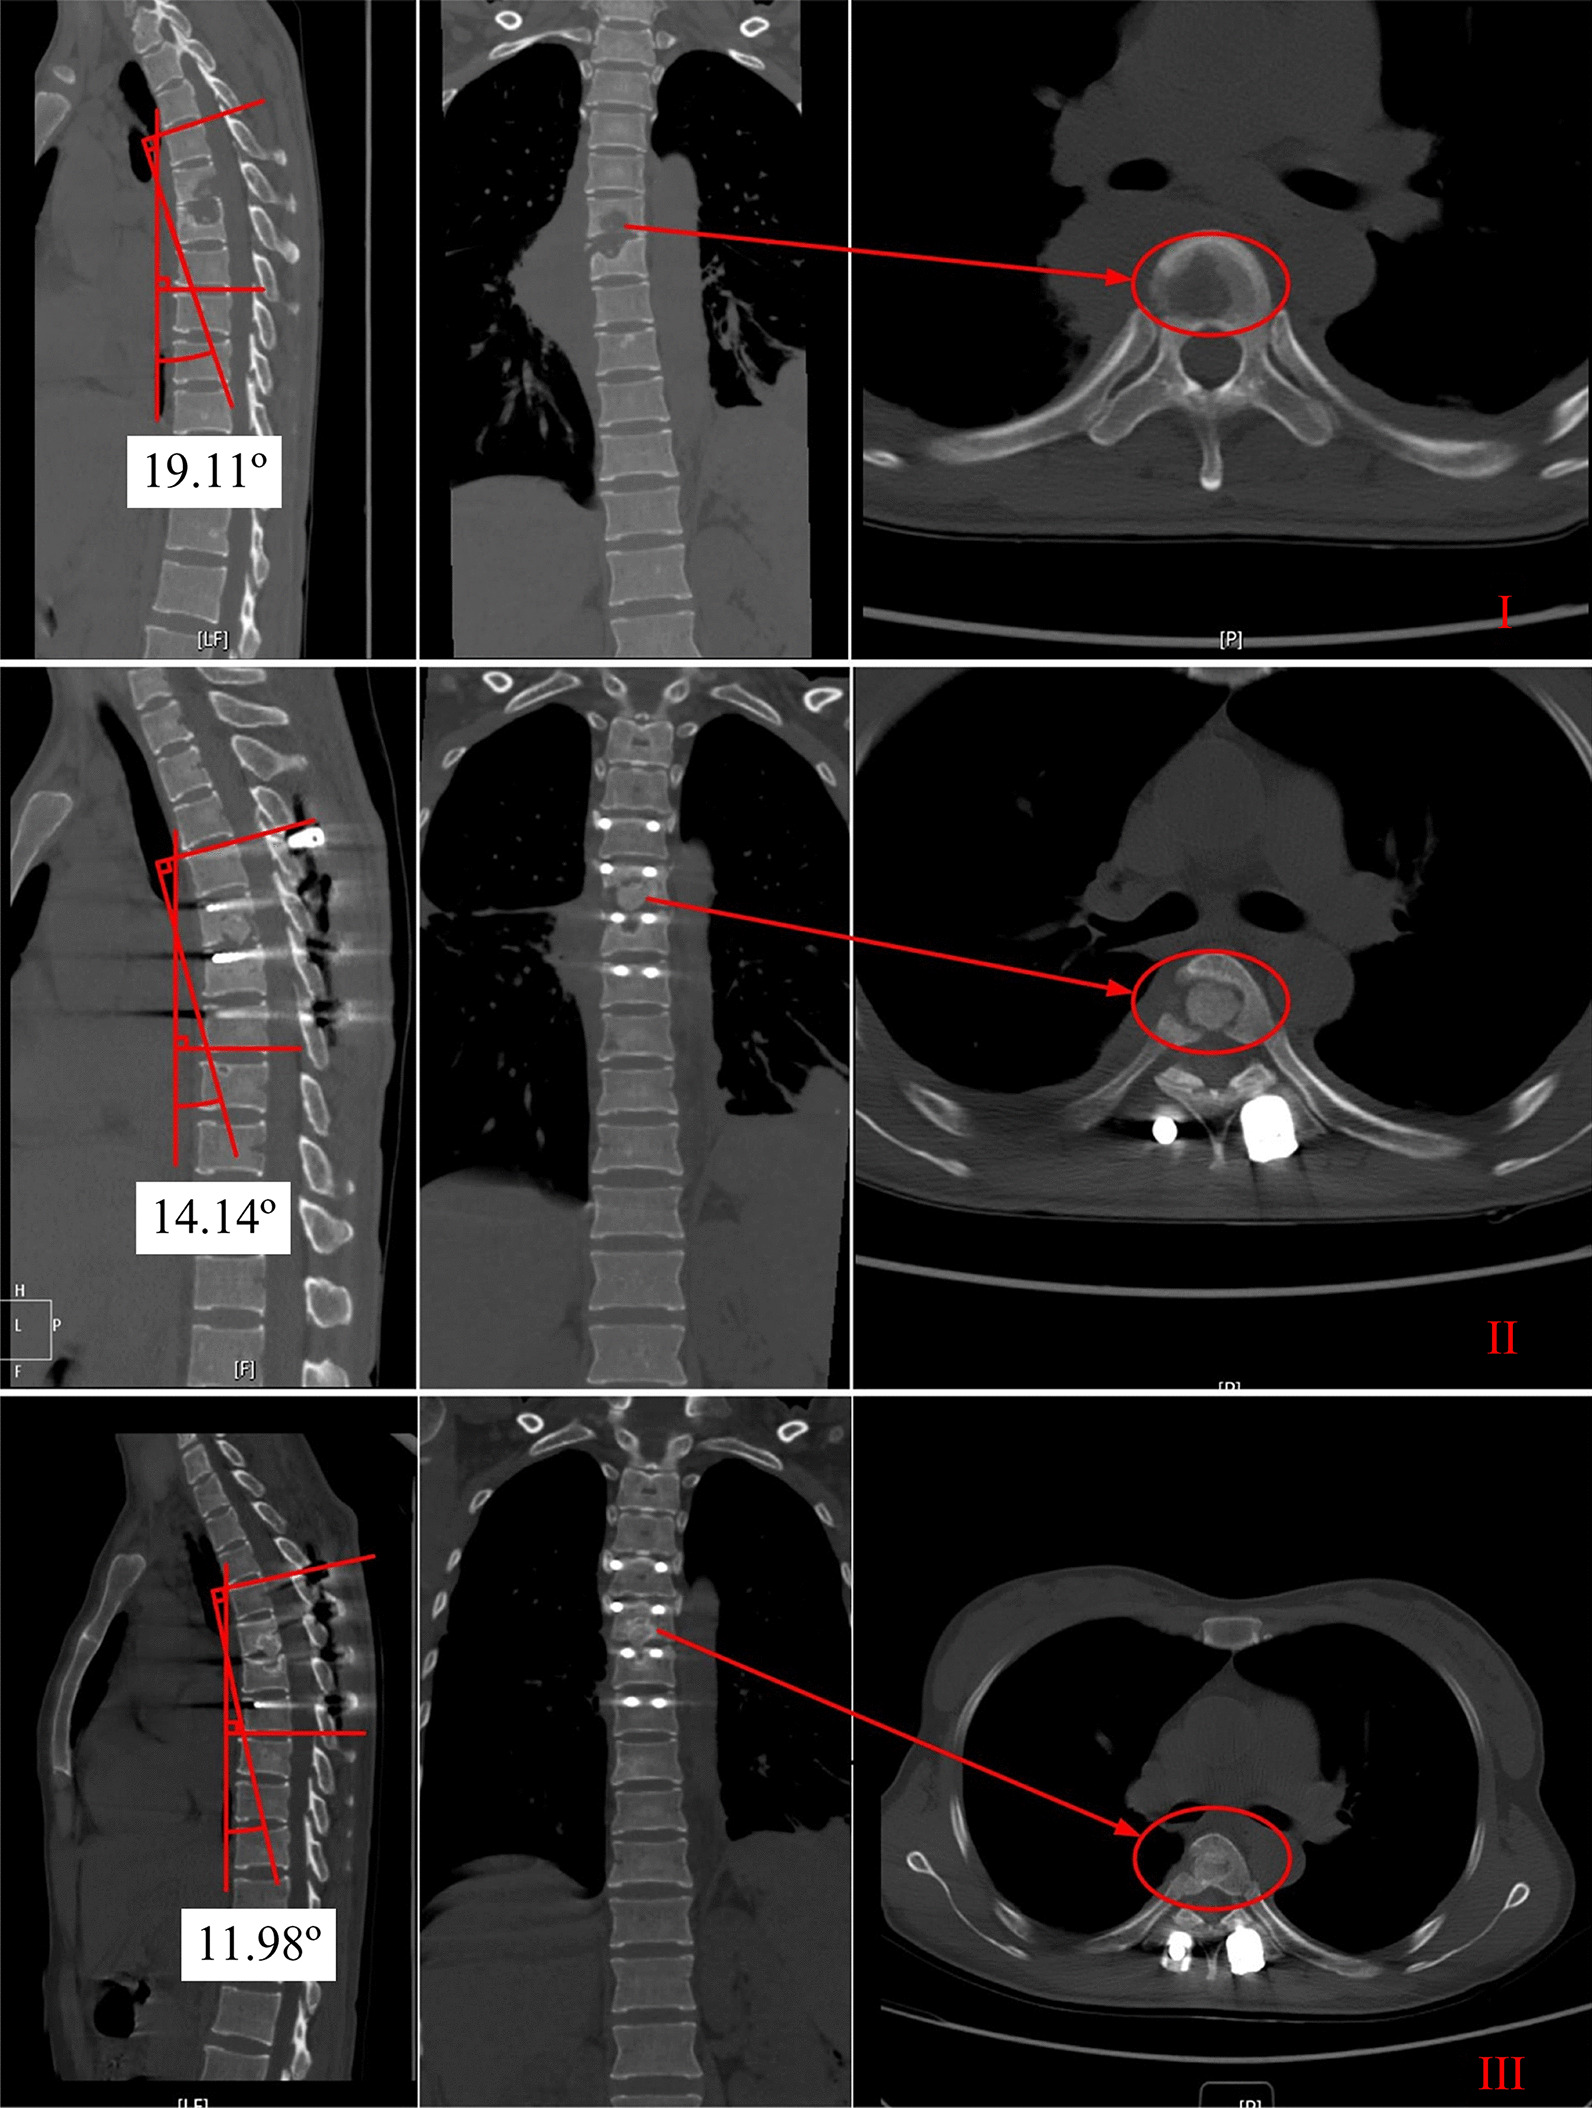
Radiological examinations of a 37-year-old female patient. (I) The CT scan at admission showed severe bone destruction and bone defect. (II) The first postoperative CT showed that the lesion had been completely cleared. (III) The 6-month post-surgery follow-up showed completed bone fusion with no loss of the thoracic angle in the patient
